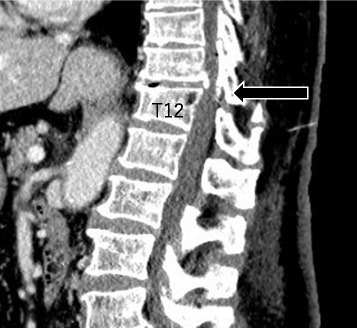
Sagittal computed tomography (CT) image of the vertebrae. The tip of the epidural tube was present at narrowest area of the spine (*arrow*)
